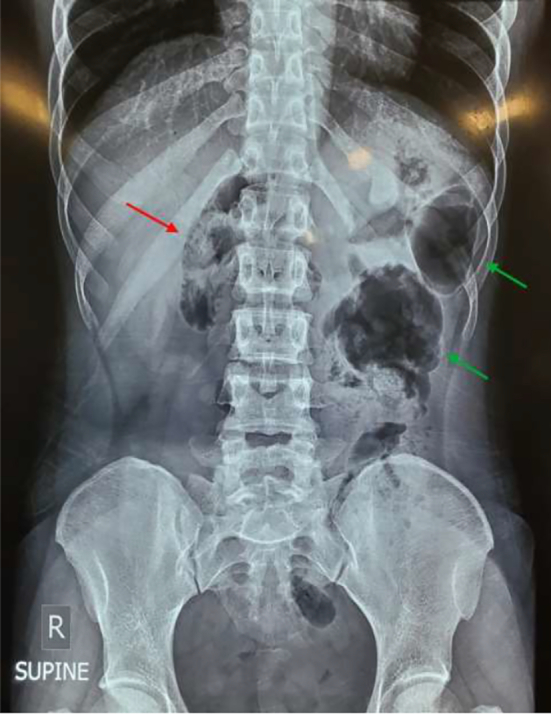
Supine plain abdominal X-ray showing caecal shadow in epigastrium (red arrow), and large bowel loops localized on the anatomical left (green arrows). (For interpretation of the references to color in this figure legend, the reader is referred to the web version of this article.)
